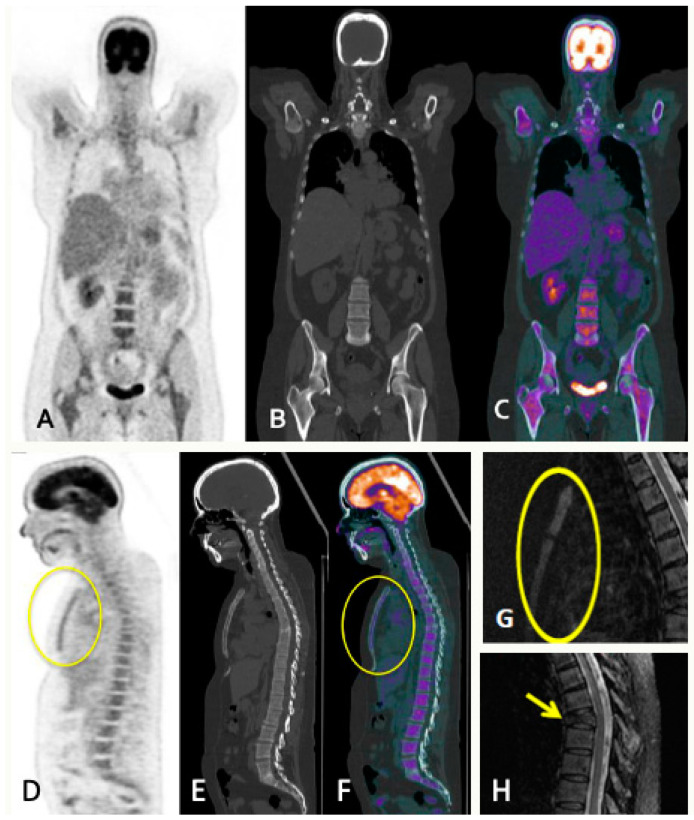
A 56-year-old female with SMM presenting lumbar pain resistant to analgesia. PET/CT images in coronal (A–C) and sagittal (D–F) show diffuse MM involvement of the bone marrow with intense metabolic activity in the axial and peripheral skeleton, and sternal involvement (circles). Spine STIR sagittal sequence (G,H) showing increased bone marrow sternal signal (circle), concordant with FDG-PET/CT findings and burst fracture in T7 vertebral body with slight posterior wall retropulsion (arrows) poorly visualized in the FDG-PET/CT study because of its lower resolution and absence of metabolic activity.
